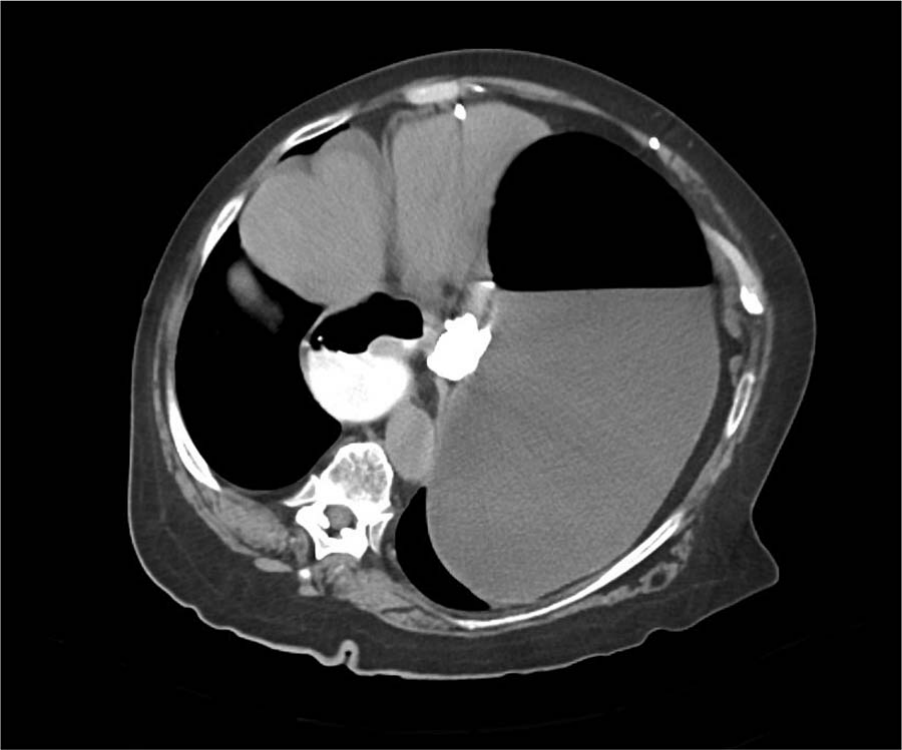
CT axial view—contrast media in distal esophagus did not pass to the stomach.
